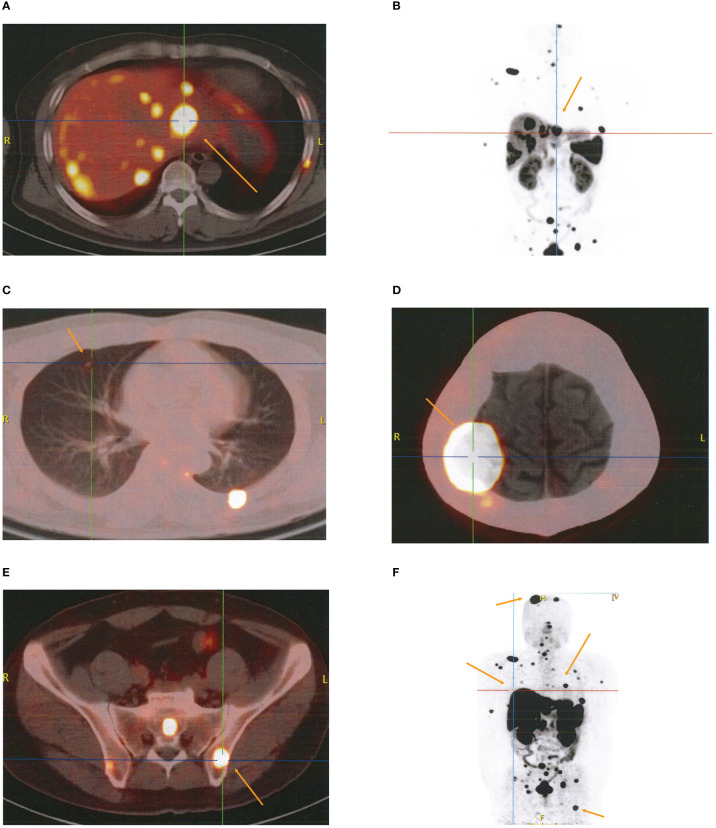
The results of ^18^F-DOTATATE PET/CT for metastatic localizations of paragangliomas. (A–C) Multiple high uptake localizations in liver (A, B) and lung (C). (D, E) High uptake localizations with osteolytic bone destruction of right parietal skull bone (D) and ilium (E). (F) Whole-body SPECT/CT imaging.
